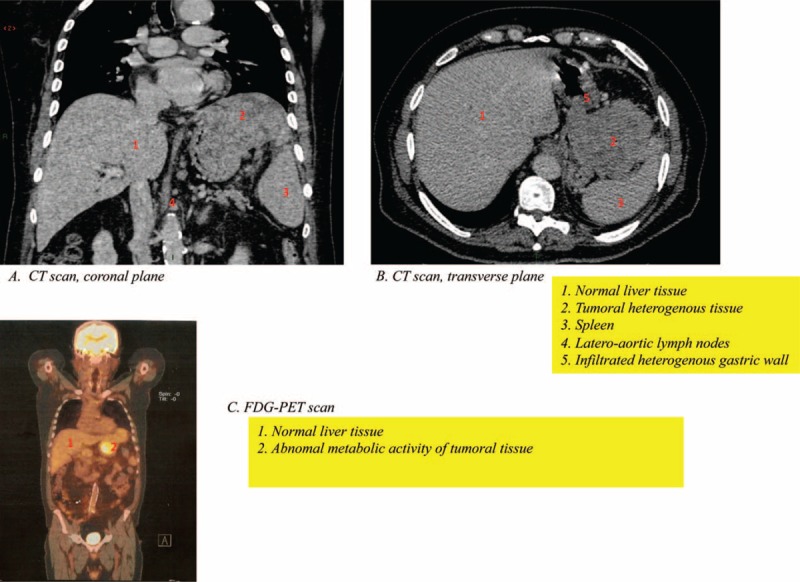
Imaging at diagnosis showing at CT scan (A and B) a large heterogeneous tumor measuring 10 × 8 mm initially the origin was taken for a gastric lesion. The tumor extends to the diaphragm, the splenic hilum, and lymph nodes. The liver seems to be normal with no evident lesions. The positron emission tomography scan showed abnormal metabolic activity located near the liver and the spleen hilum (C). CT = computed tomography.
